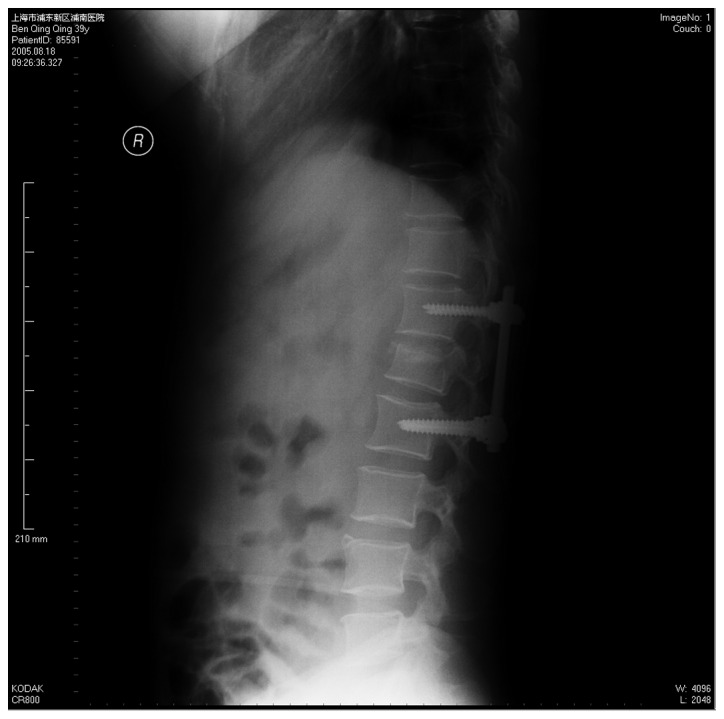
Lateral radiograph following dual-plane fixation of an L1 fracture.
